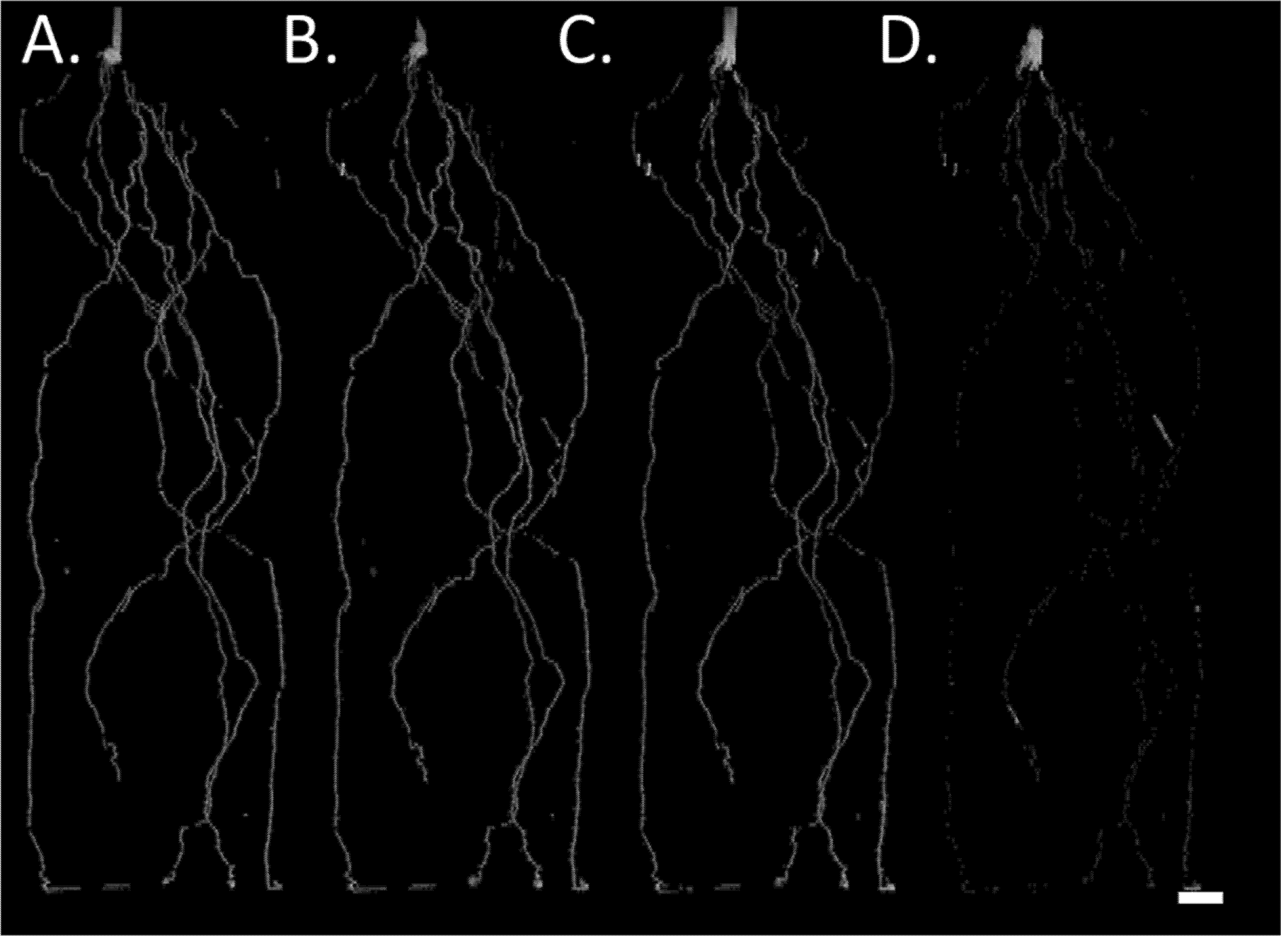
MRI signal intensity development of barley seminal root over time. (A) Complete root system at 12 DAG, (B–D) root system 19, 27, and 35 DAG; only roots already present at 12 DAG are shown. Plants were grown in a cylindrical mesocosm (30 cm × 8 cm). Reductions in root signal intensity occur in older root segments but not in for example, the stem which functions as max intensity reference. For image D the shoot was removed prior to the MRI experiment. Resolution 0.5 × 0.5 × 1.0 mm^3^, Scale bar = 2 cm.
